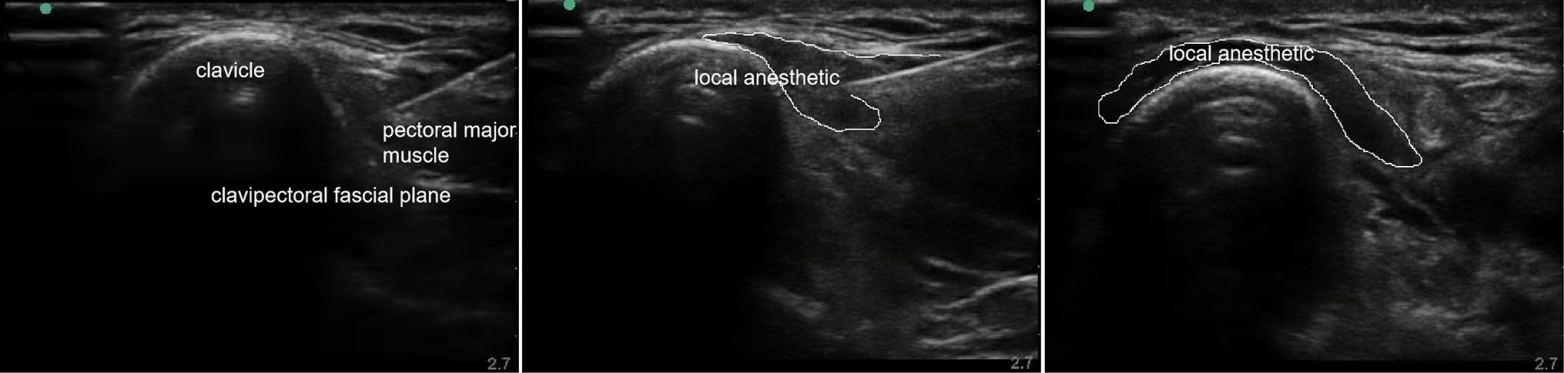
Ultrasound image of clavipectoral fascial plane block
